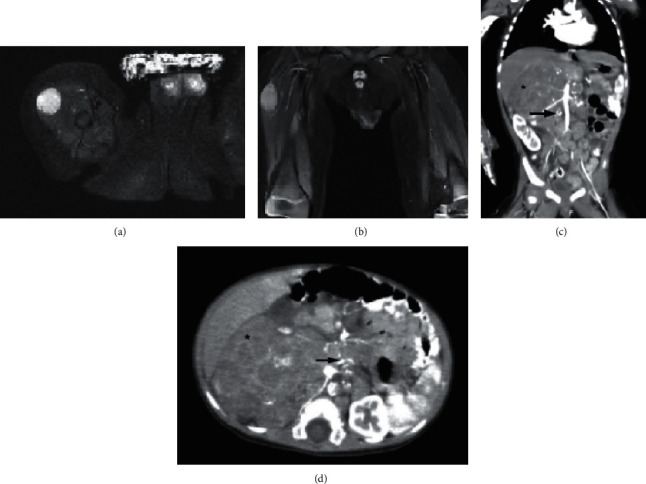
Initial axial T2-weighted MRI revealed the soft tissue lesion with a hyperintense signal in axial (a) and coronal (b) views. Abdominopelvic CT scan coronal (c) and axial (d) views show a heterogenic mass (black stars) in the subhepatic along with paraaortic calcified lymph nodes (black arrows).
